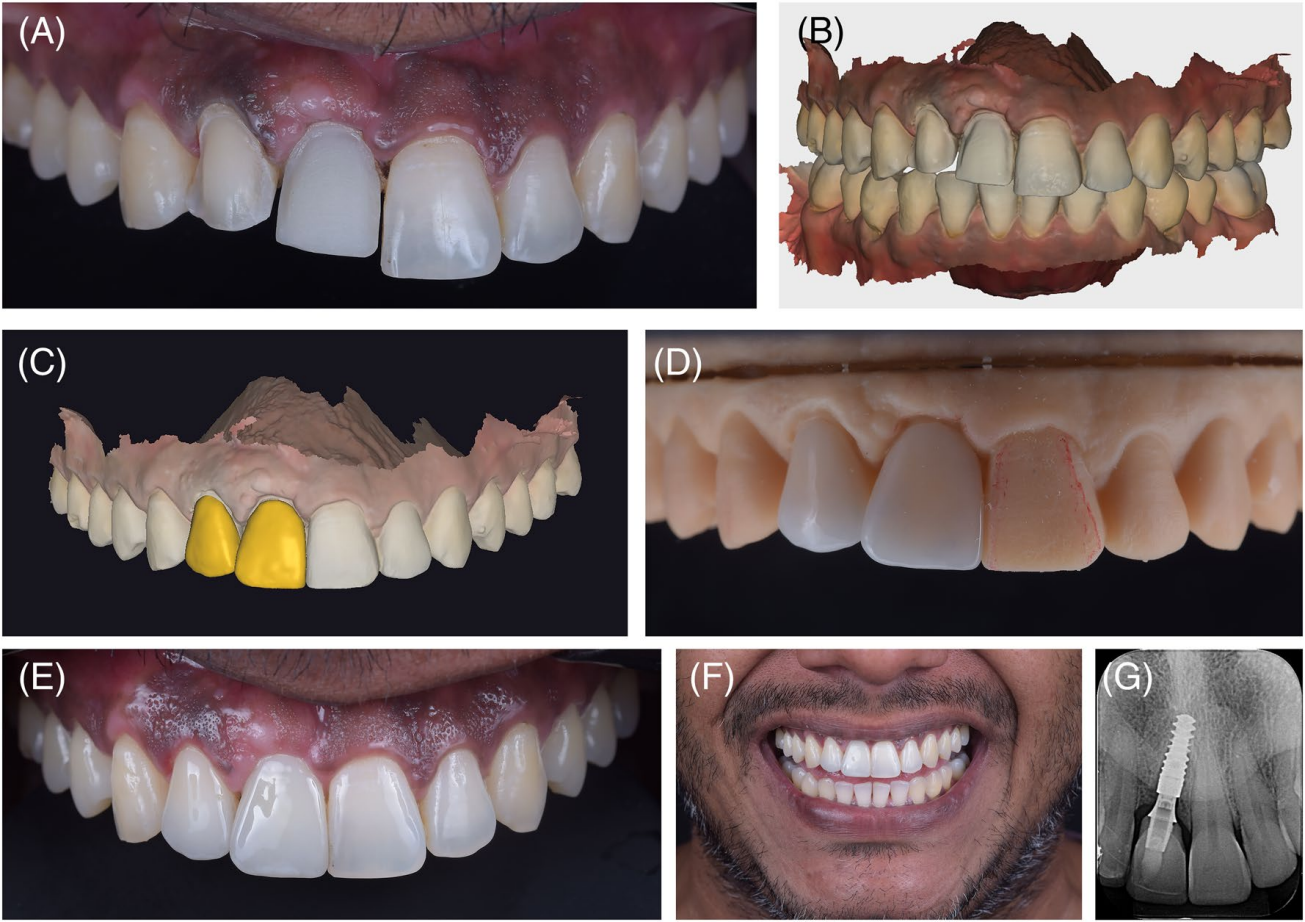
(A) Preparation of tooth 12 for a laminate veneer and try‐in of the custom coping over the Variobase abutment on tooth 11; (B) Scan of the tooth preparation 12 and the custom abutment on tooth 11; (C) CAD of the laminate veneers; (D) ceramic veneers on the model; (E) intraoral view of the laminate veneers; (F) frontal view of the smile with the laminate veneers; (G) periapical radiograph at the completion of treatment.
